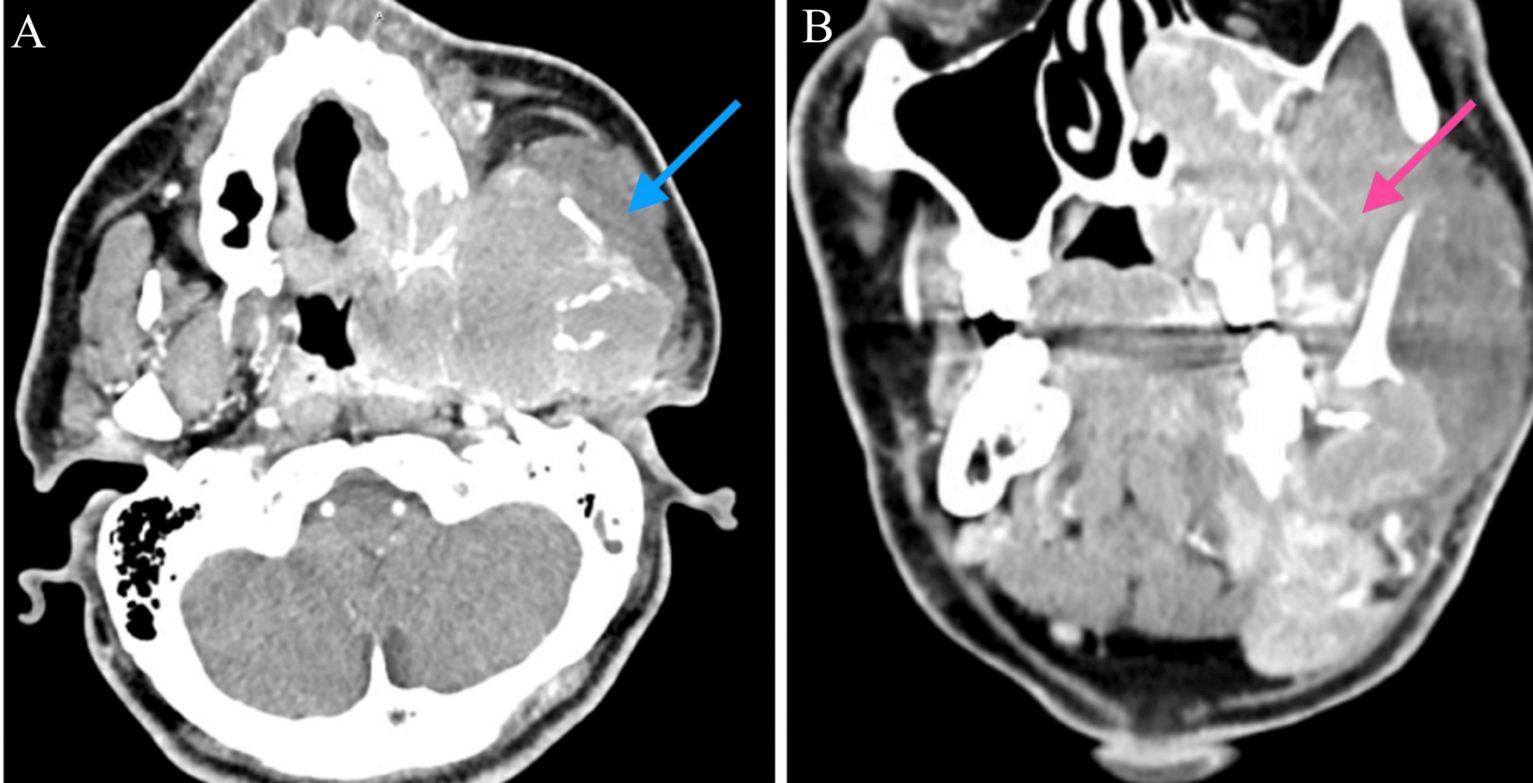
CECT of the face demonstrating extensive destructive left mandibular mass at initial presentation (A) Axial view shows a large heterogeneous soft-tissue mass centered in the left mandibular region with aggressive local extension (blue arrow). (B) Coronal view demonstrates the superior–inferior extent of the lesion with involvement of adjacent maxillofacial structures (pink arrow), consistent with extranodal diffuse large B-cell lymphoma with locally destructive features. CECT: contrast-enhanced computed tomography
